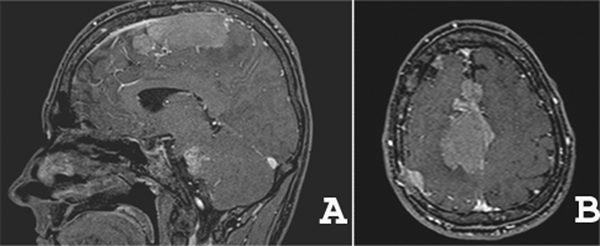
Sagittal (A) and axial (B) T1 weighted contrast enhanced MR images showing multiple dural based homogeneous enhancing masses, suggesting the diagnosis hypothesis of multiple meningiomas.
